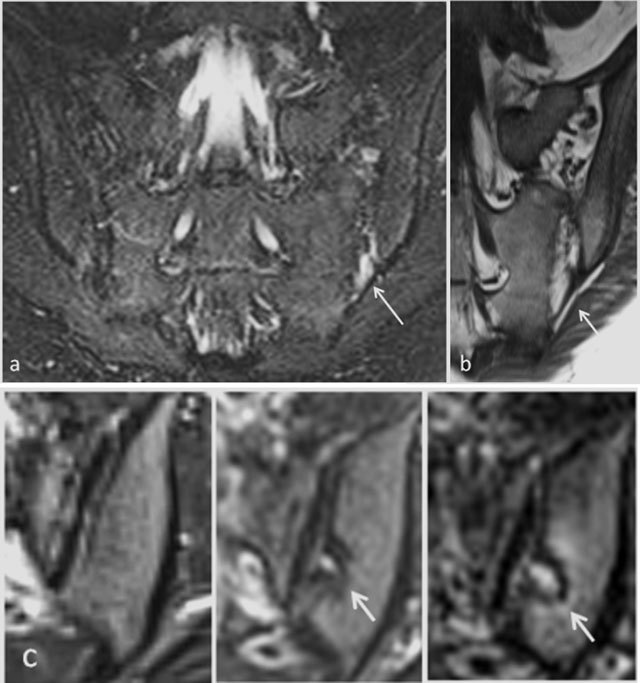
a. Paracoronal STIR images in a 16-year-old girl with enthesitis-related arthritis demonstrates a left-sided enthesitis of the retroarticular interosseous ligaments as only finding at the first MRI of the sacroiliac joints. b. T1 image demonstrates that the high signal is seen in the retroarticular fat tissue and not in the cartilaginous part of the SI joint. c. Paracoronal STIR image at first MRI, two and three years later. Follow-up MRI in this patient shows formation of a small erosion at the iliac side of the left sacroiliac joint, evolving to a large erosion three years later.
